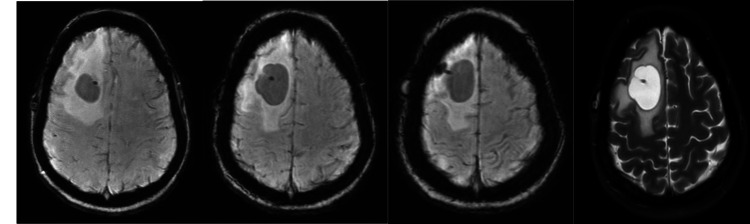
Initial brain MRI showing an approximately 4-cm intraparenchymal cyst in the right frontal lobe with surrounding vasogenic edema Minimal ring enhancement around the cyst is seen.
